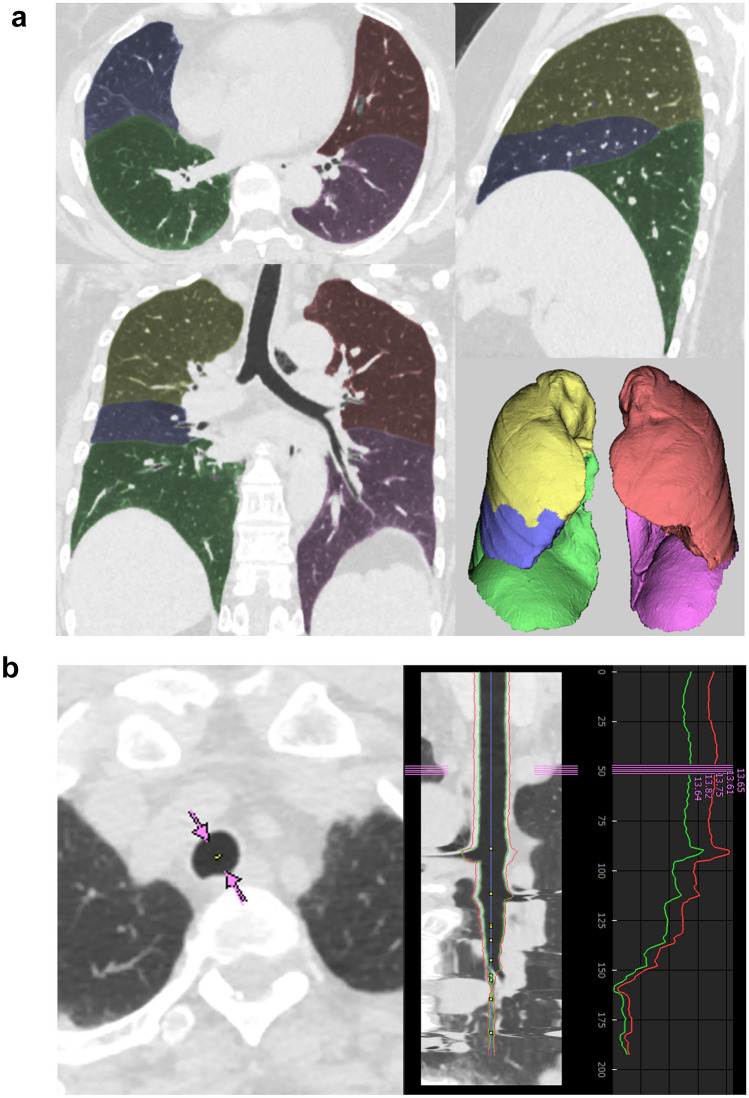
Evaluation of the a lung volume and b tracheal diameter using a software. a The right upper lobe, right middle lobe, right lower lobe, left upper lobe, and left lower lobe were segmented and highlighted with yellow, blue, green, red, and pick, respectively. b Trachea at the thoracic inlet is annotated with pink arrows in the left CT image. Inner lumen and outer boundary are shown with green and red lines, respectively. Inner lumen diameter is also displayed as pink numerals
